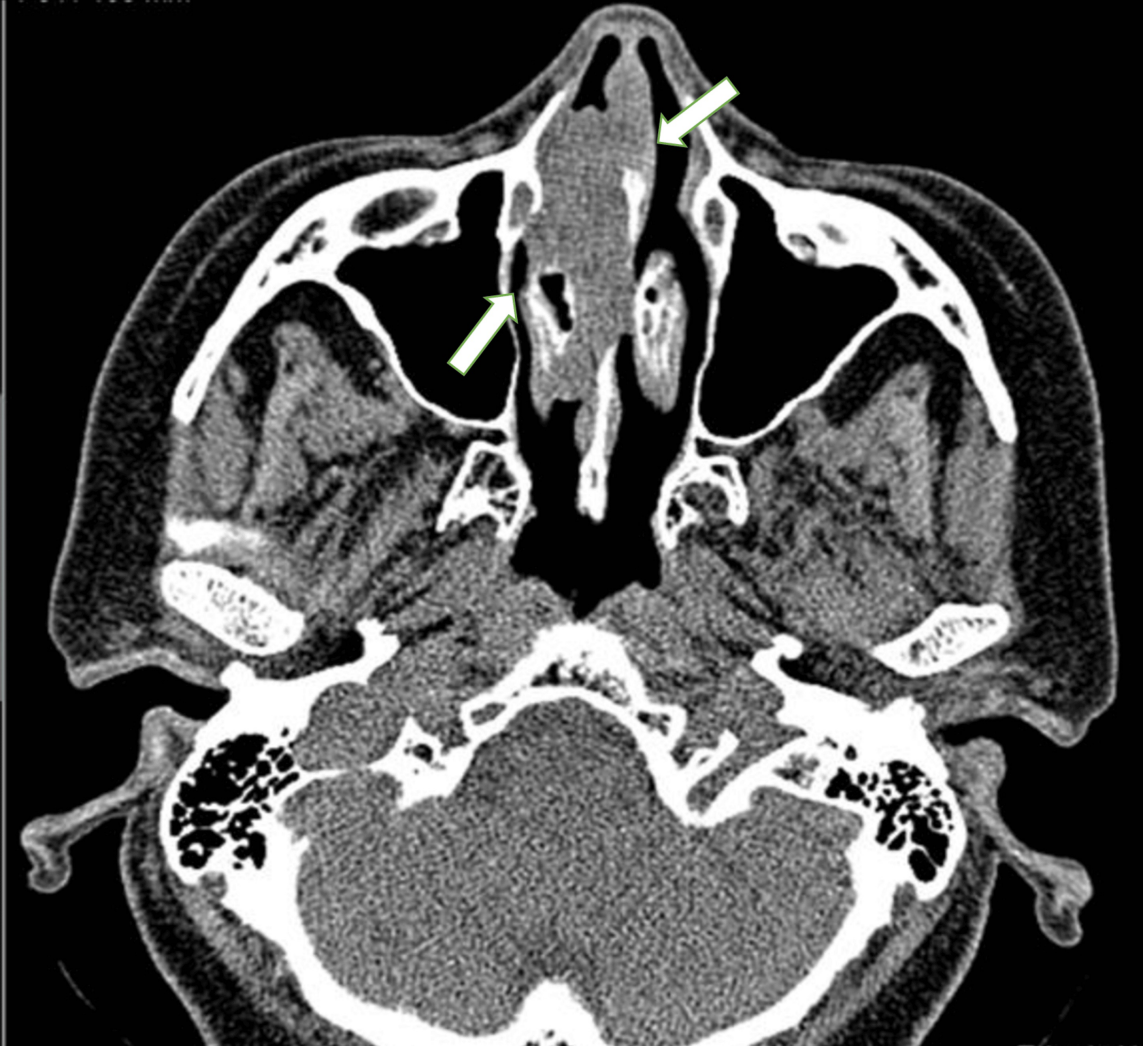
Plain CT of the nose and paranasal sinus: axial view showing an isodense mass in the right nasal cavity with erosion of the nasal septum. CT: computed tomography
